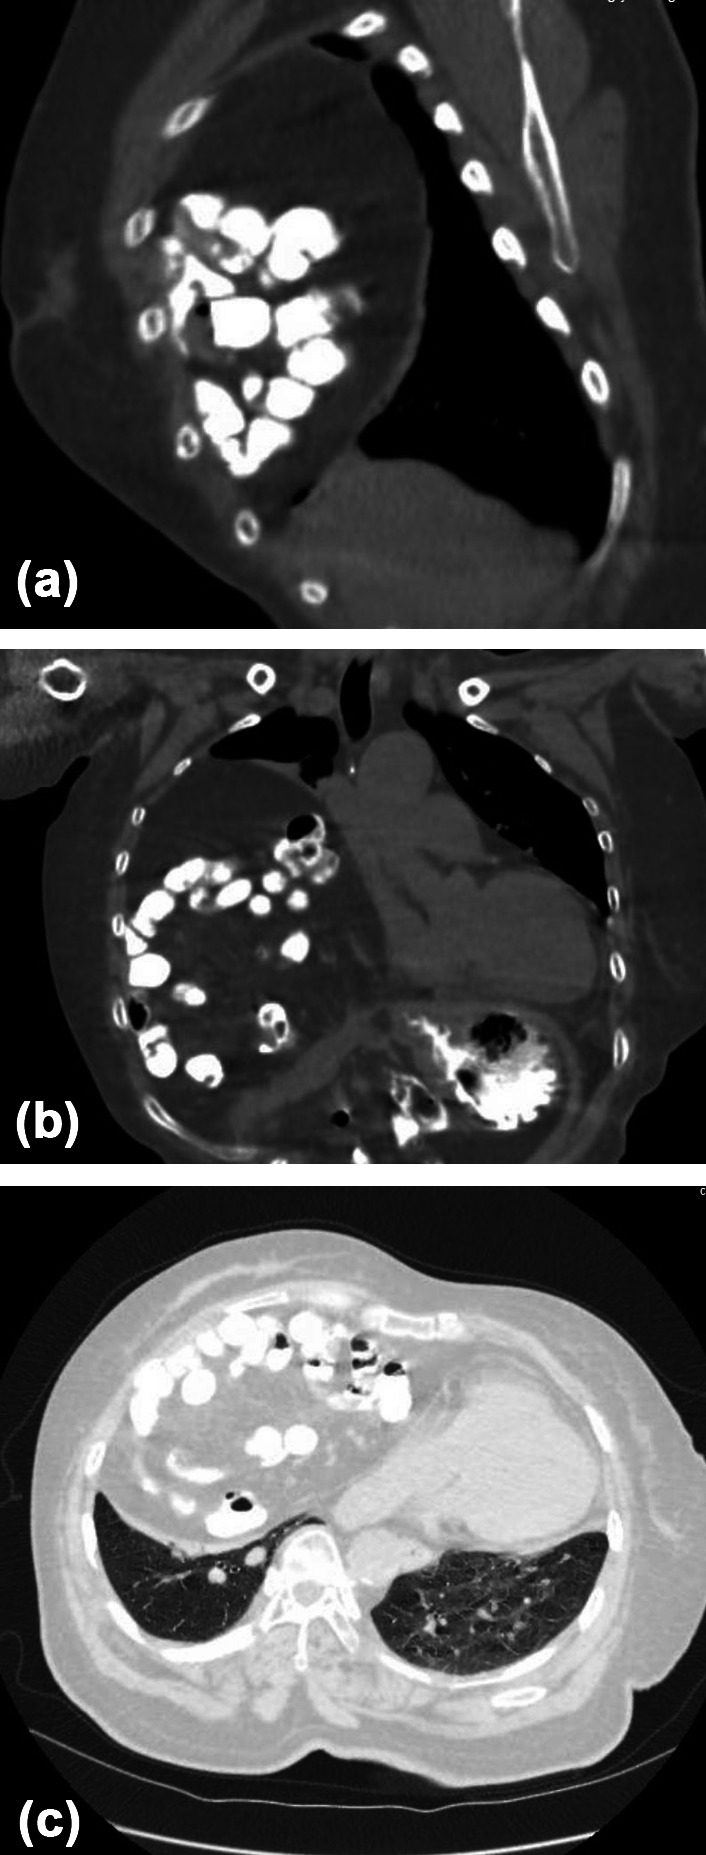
The hernia contents have entered the thoracic cavity.(a)Sagittal CT image.(b)Coronal CT image.(c)Axial CT image.
